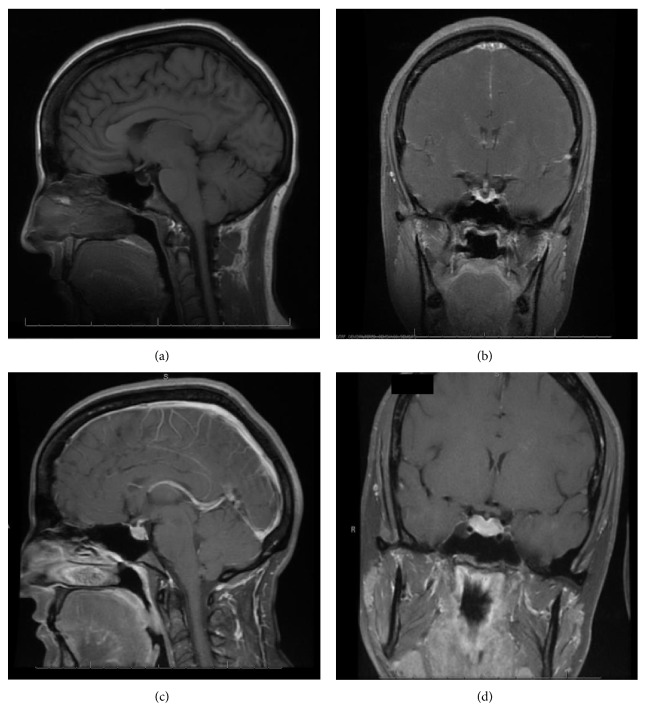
Case MR images. Sagittal (a) and coronal (b) unenhanced images of the pituitary, revealing a slightly atrophic pituitary gland. Two months later, at presentation, sagittal (c) and coronal (d) postcontrast images reveal an enlarged pituitary size with diffuse enhancement of the pituitary and infundibulum without focal lesion.
